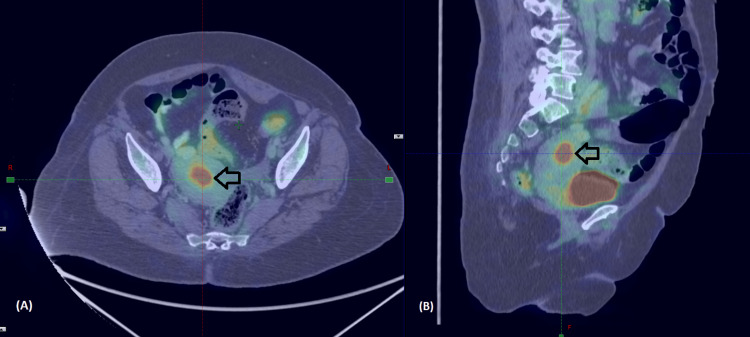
Axial (A) and sagittal (B) sections of PET-CECT showing intrauterine lesion PET-CECT: Positron emission tomography and contrast-enhanced computed tomography
